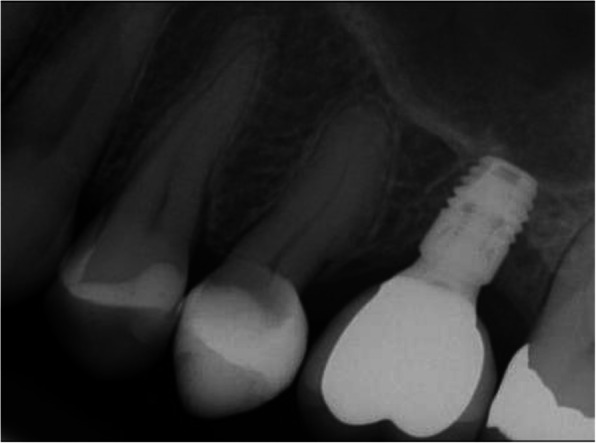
Periapical radiograph of a short implant at baseline
